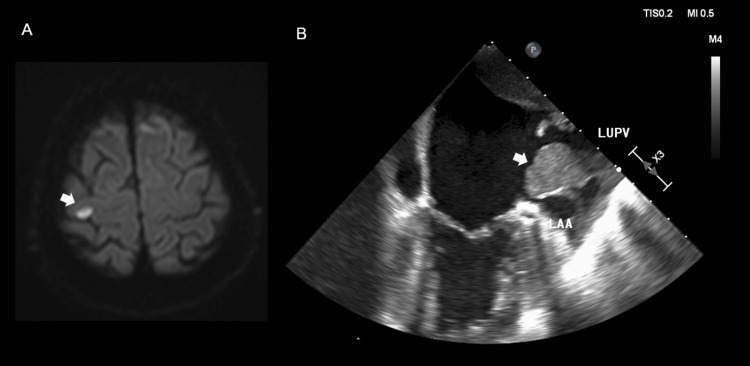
Brain MRI and transesophageal echocardiography. (A) Brain MRI shows hyperintensity in the right precentral knob on diffusion-weighted imaging (arrow) with decreased apparent diffusion coefficient (not shown). (B) Transesophageal echocardiography shows tumor invasion into the left atrium and a tumor mass with mobility (8 × 6 mm, arrow) at the left pulmonary vein ostium. The arrow indicates the mass. LUPV: left upper pulmonary vein; LAA: left atrial appendage
